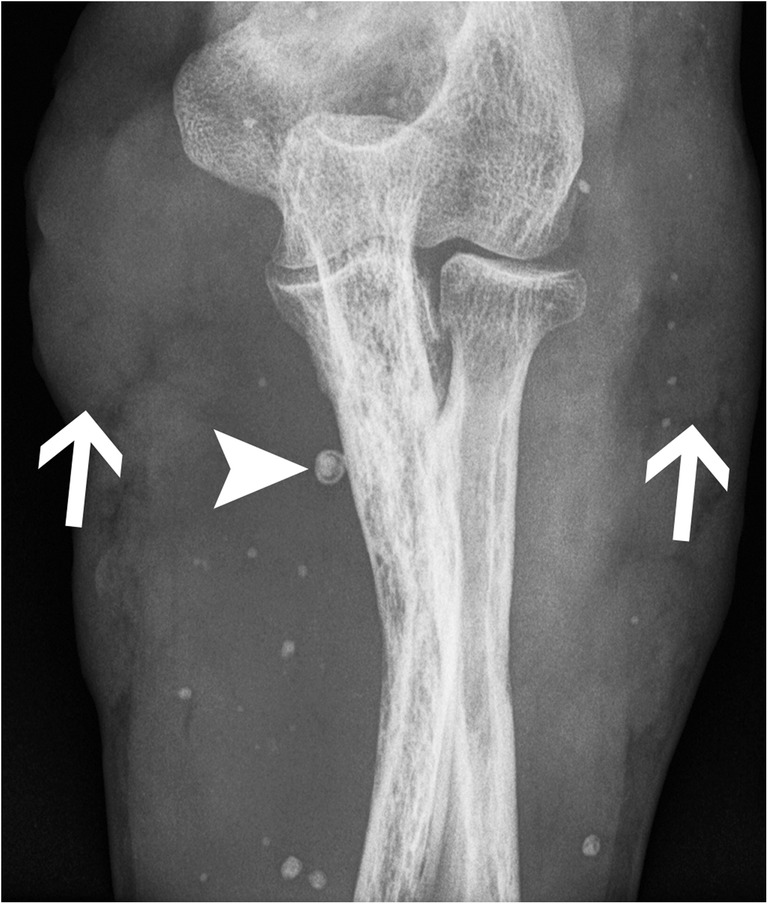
Phleboliths. Anteroposterior radiograph of the elbow shows phleboliths in the soft tissues with the characteristic central lucency (arrowhead) in a patient with a venous malformation of the upper extremity. Note multifocal soft tissue masse effect that corresponds to the diffuse venous malformation (arrows)
